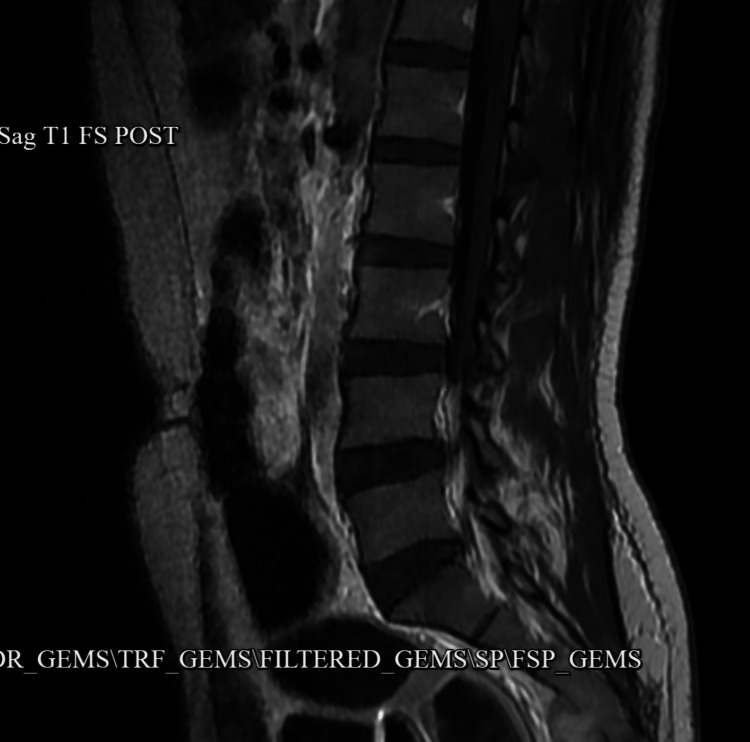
MRI of the lumbar spine was unremarkable MRI: magnetic resonance imaging.
